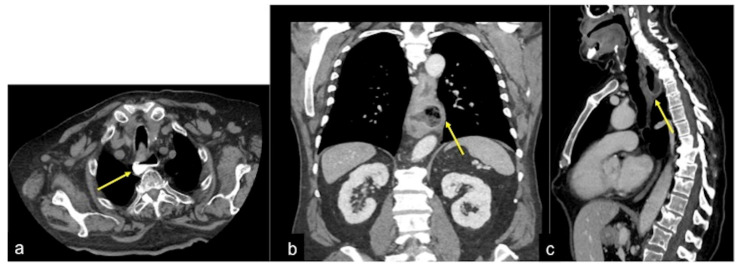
Oesophageal diverticula. Axial (a), coronal (b) and sagittal (c) images of the CTA multiphasic study show a wall protrusion in the middle oesophagus with mucosal hyperaemia as a possible site of bleeding (a–c, arrow).
